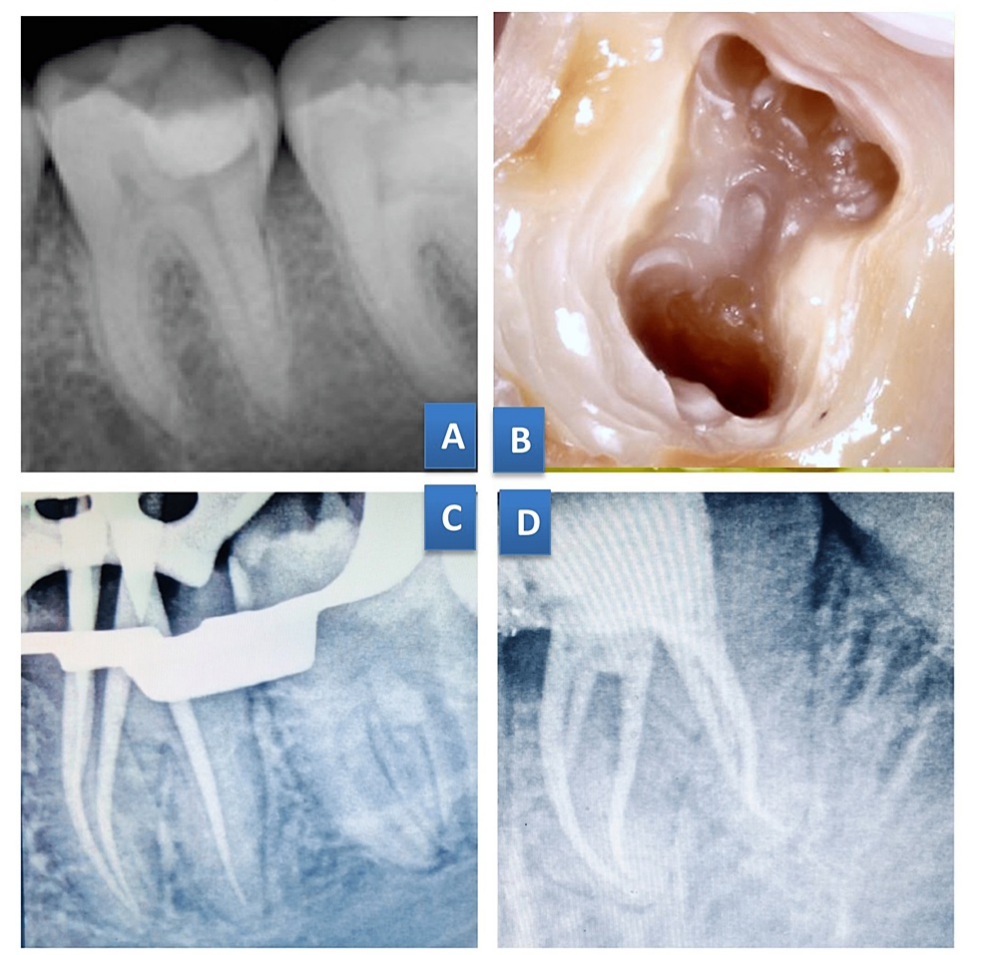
Case 2. Middle mesial canal merged with mesiolingual canal at the middle third region in 46 (A) Preoperative radiograph;  (B) Access cavity preparation; (C) Master cone radiograph; (D) Post-obturation radiograph
